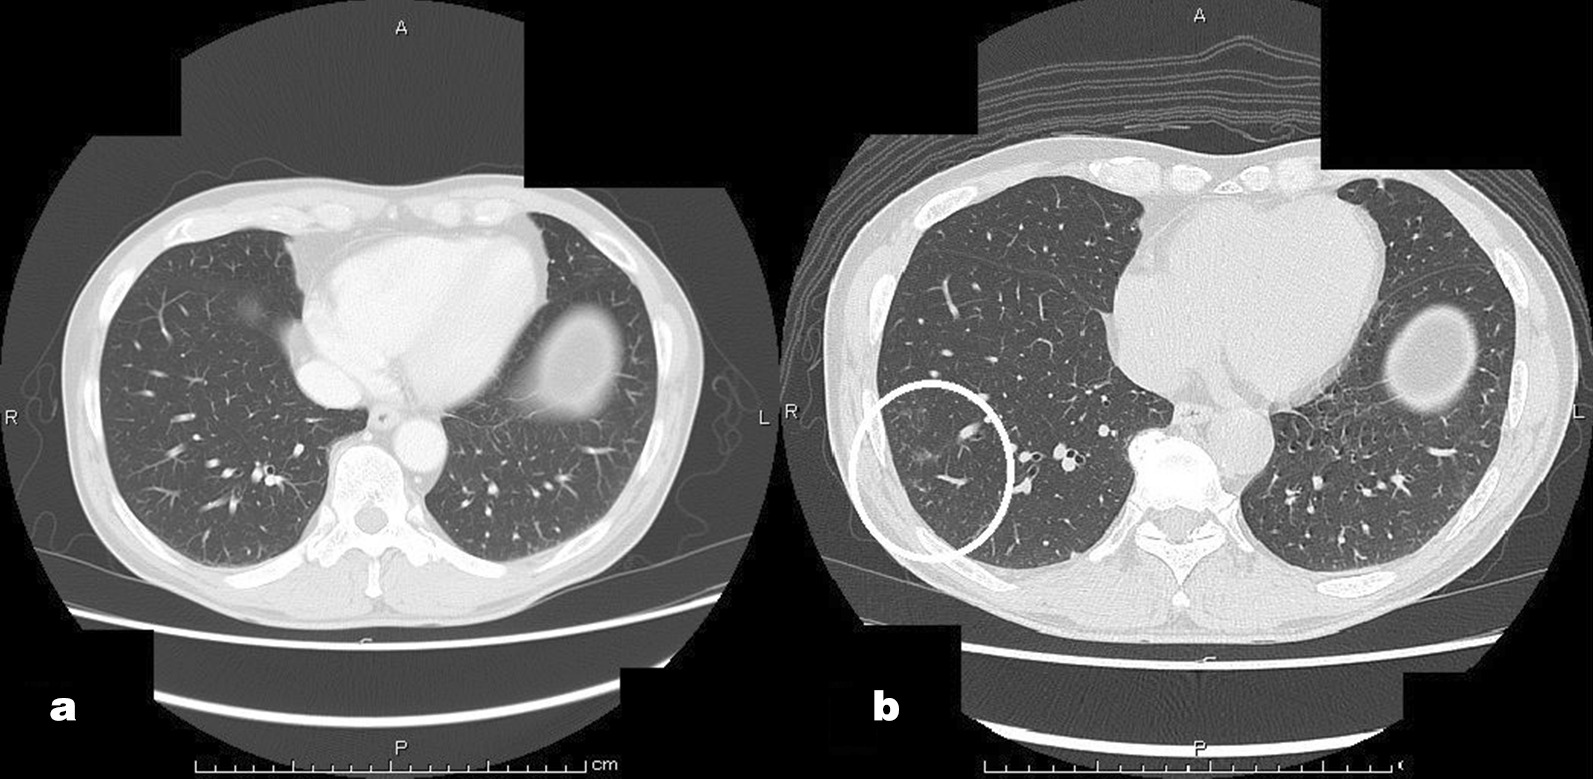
Chest computed tomography scan findings. The images show a no abnormal findings 2 months before the operation and b a ground glass opacity in the periphery of the right lower lobe 2 days before the operation (white circle)
